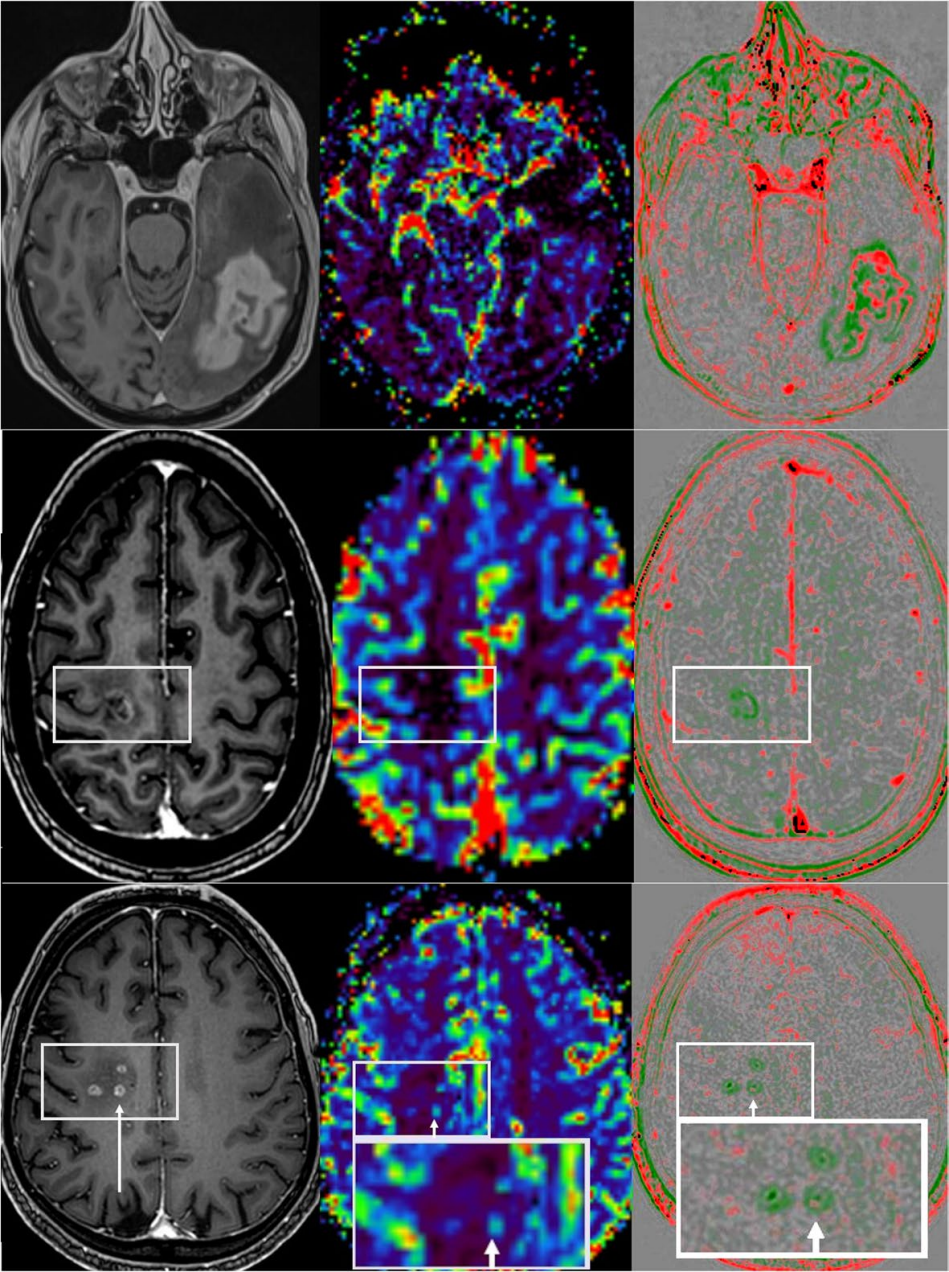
Glioblastoma therapy assessment. Top: 200% volume progress in 6-week follow-up. MR perfusion (middle) showed no local hyperperfusion. Rapid wash-out (right) revealed areas of increased rapid wash-out (red). Middle: Typical post-therapeutically changes without hyperperfusion and wash-out, but wash-in (green). Bottom: Radiation necrosis (3-month follow-up was stable) with less active tumor cells with bubble-like appearance of small contrast enhancing lesions and minimal hyperperfusion (arrow) as well as minimal ring-shaped wash-outs with red dot (arrow). Left: contrast enhanced T1-weighted images; middle rCBV map of MR perfusion; right: rapid wash-out map
